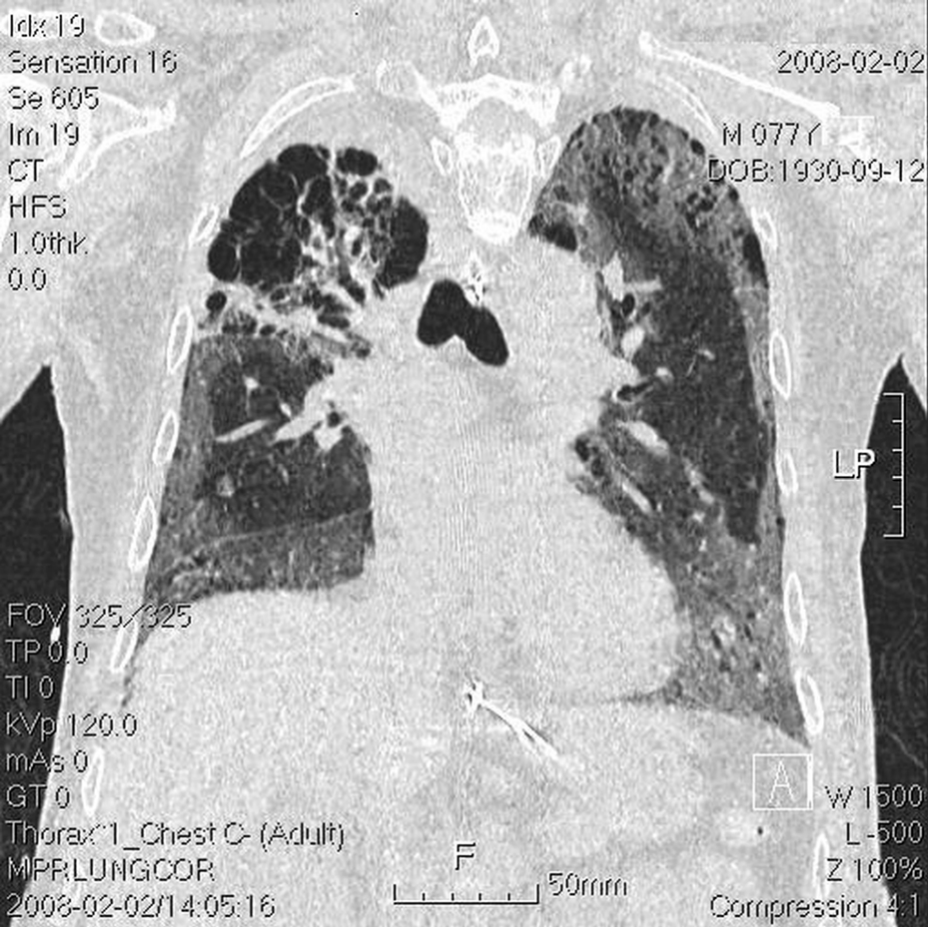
Coronal view of the whole lung field. Chest computed tomography (CT) done post intubation shows ground-glass opacities, blebs confined to the right upper lung apex, and diffuse ground-glass attenuation, blebs in the marginal areas, airspace consolidation, and fibrosis in the bilateral upper and lower lung fields.
